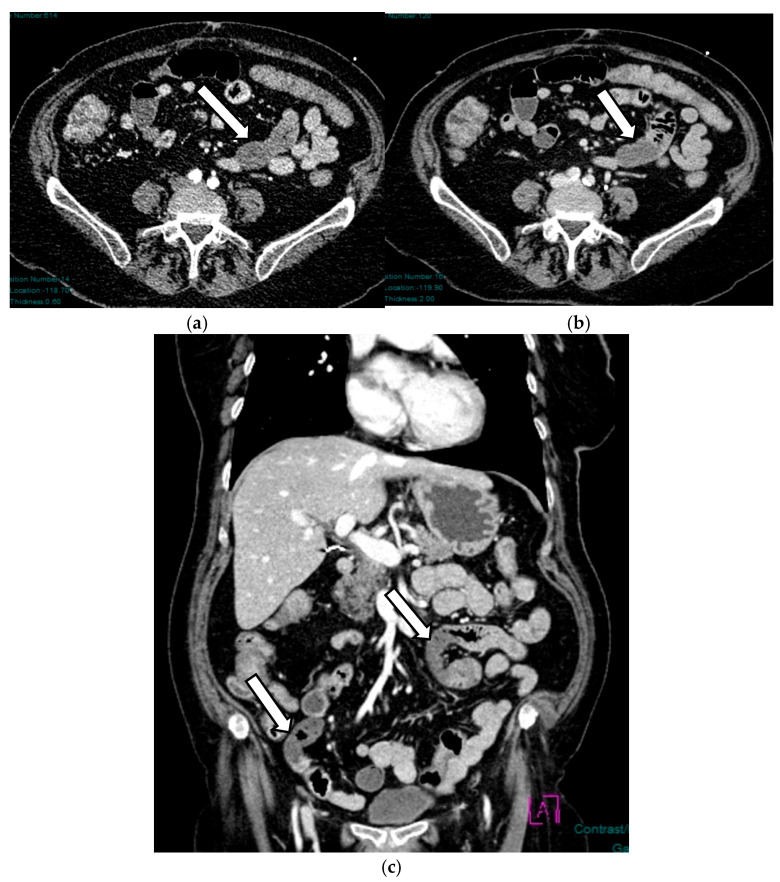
AAMI in a 64-year-old woman: axial (a,b) and coronal (c) post-contrast CT scans show some ileal loops with absent wall enhancement in the meso-hypogastric region, especially if compared to the adjacent loops (arrows).
